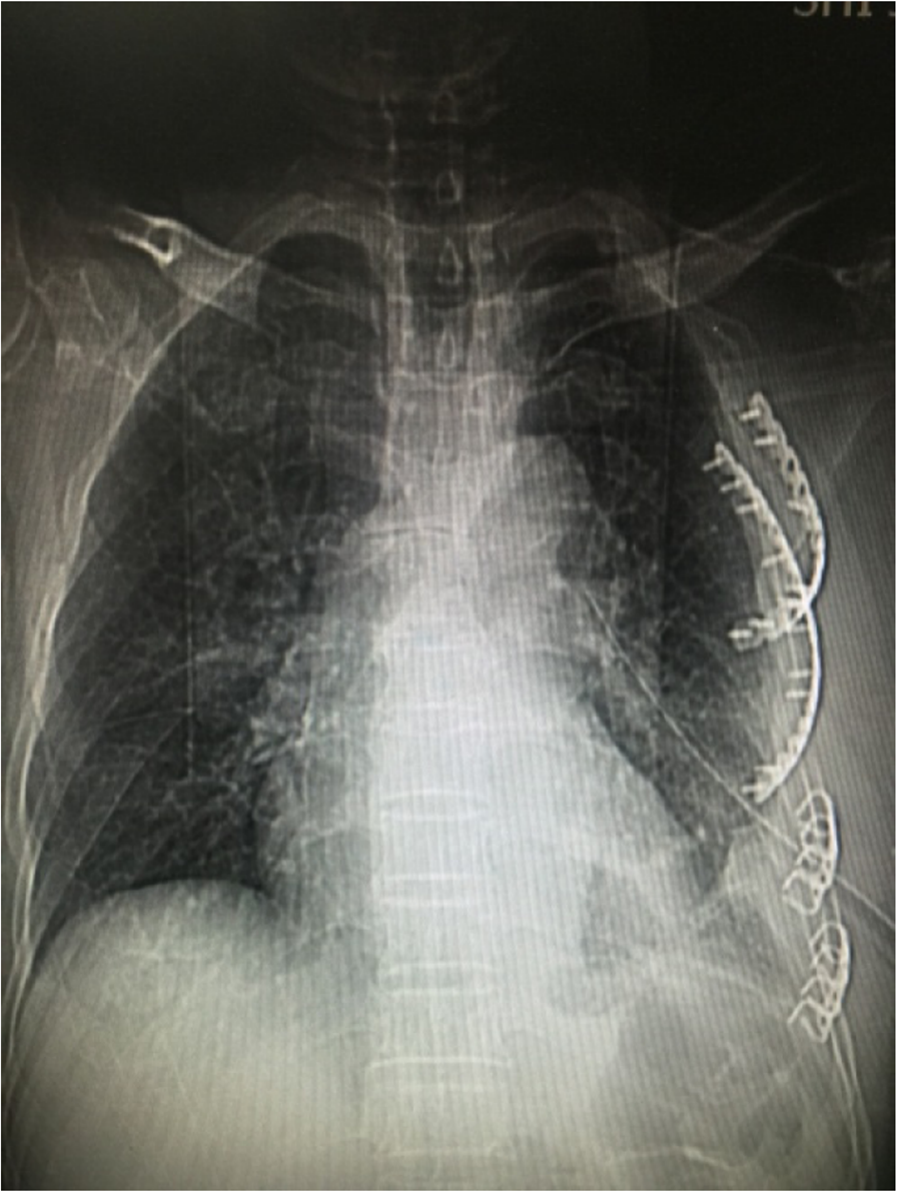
post-operation review of the chest radiograph. In post-operation review of the chest radiograph, the shape of the internal fixation is intact and completely symmetrical with the contralateral ribs
